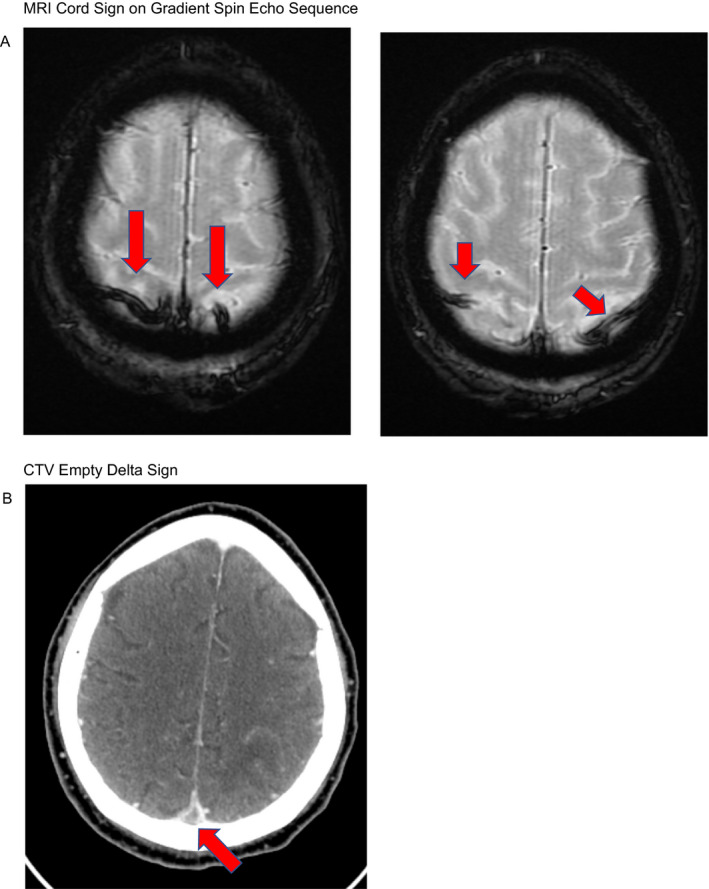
(A) MRI Cord Sign on Gradient Spin Echo Sequence and (B) CTV Empty Delta Sign.
